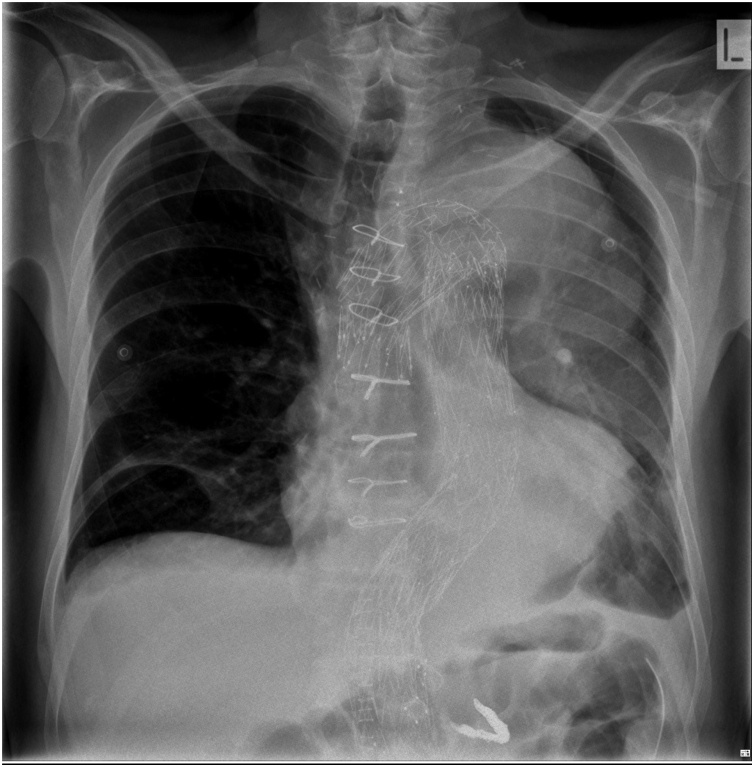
Chest x-ray post operatively demonstrating previous sternotomy wires, endovascular grafts, embolisation coils within the splenic artery and intra-abdominal drain.
